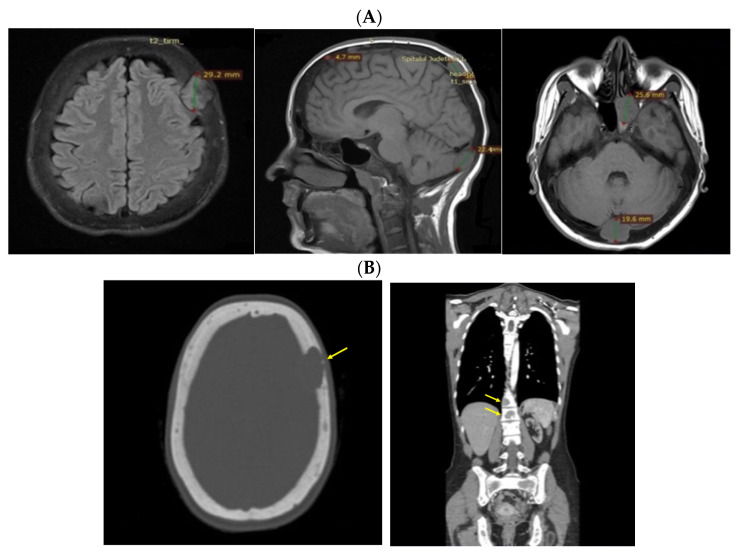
Brown tumors in an adult lady with poorly controlled renal hyperparathyroidism. (A) Native craniocerebral magnetic resonance imagery (the lesions are highlighted as well as their largest diameter). (B) Intravenous contrast computed tomography showing the mentioned lesions (yellow arrow) at the level of the skull (left) and spine (right).
